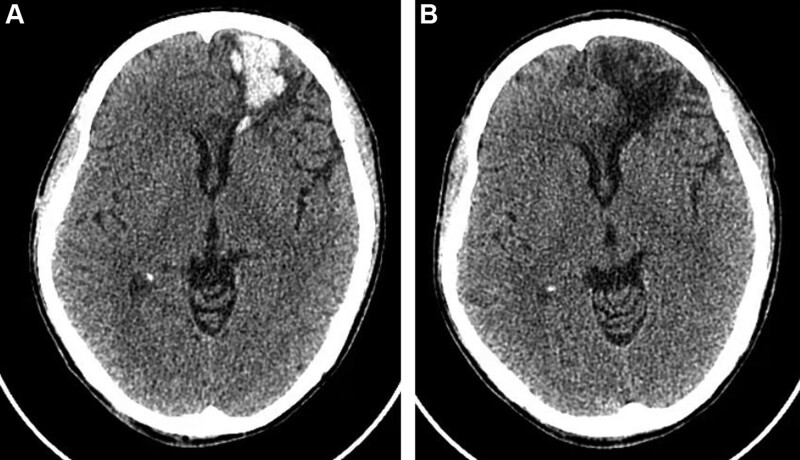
Head CT findings of left frontal brain contusion with hematoma, (A) head CT findings about 3 hours after trauma, (B) CT findings of the head at about 29 hours after trauma. CT = computed tomography.
